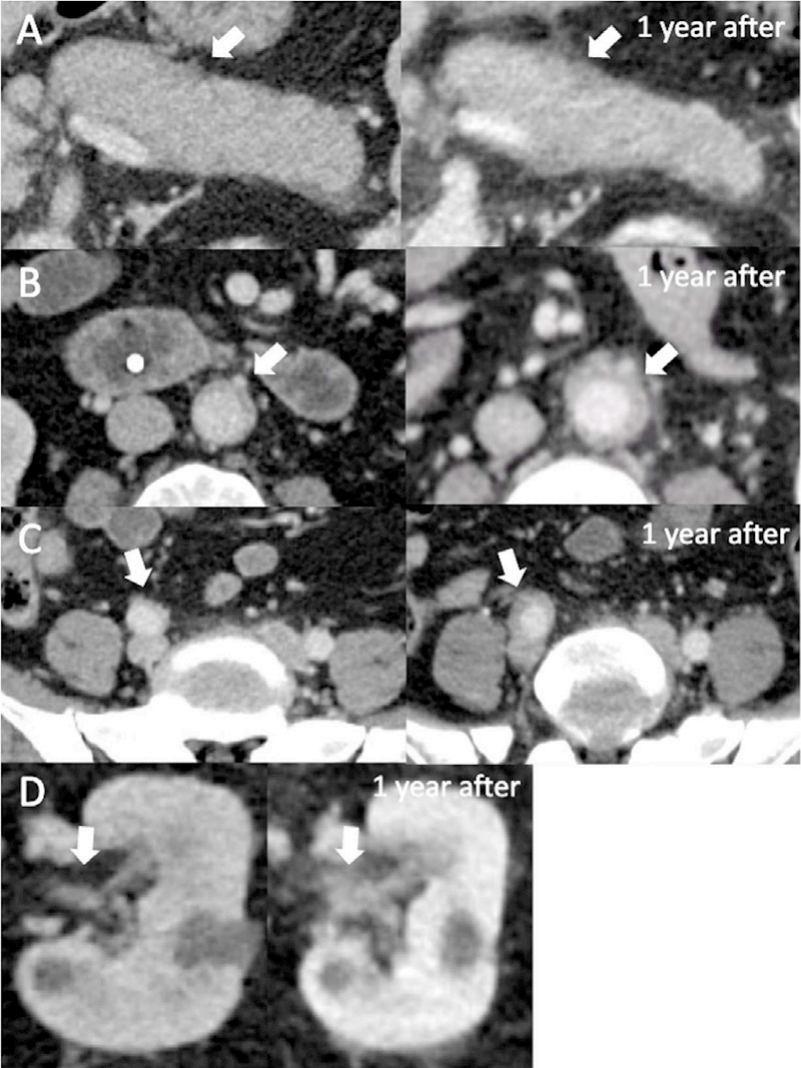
(A) Axial CT imaging dated 1 year apart with increased ill-defined halo of soft tissue attenuation surrounding the pancreas (white arrow). (B) Axial CT imaging dated 1 year apart with increased soft tissue thickening around the abdominal aorta (white arrow). (C) Axial CT imaging at the level of the pelvis with soft tissue thickening around the right iliac artery (white arrow). (D) Axial CT imaging that is dated 1 year apart with increased thickening of the left renal pelvis (white arrow).
